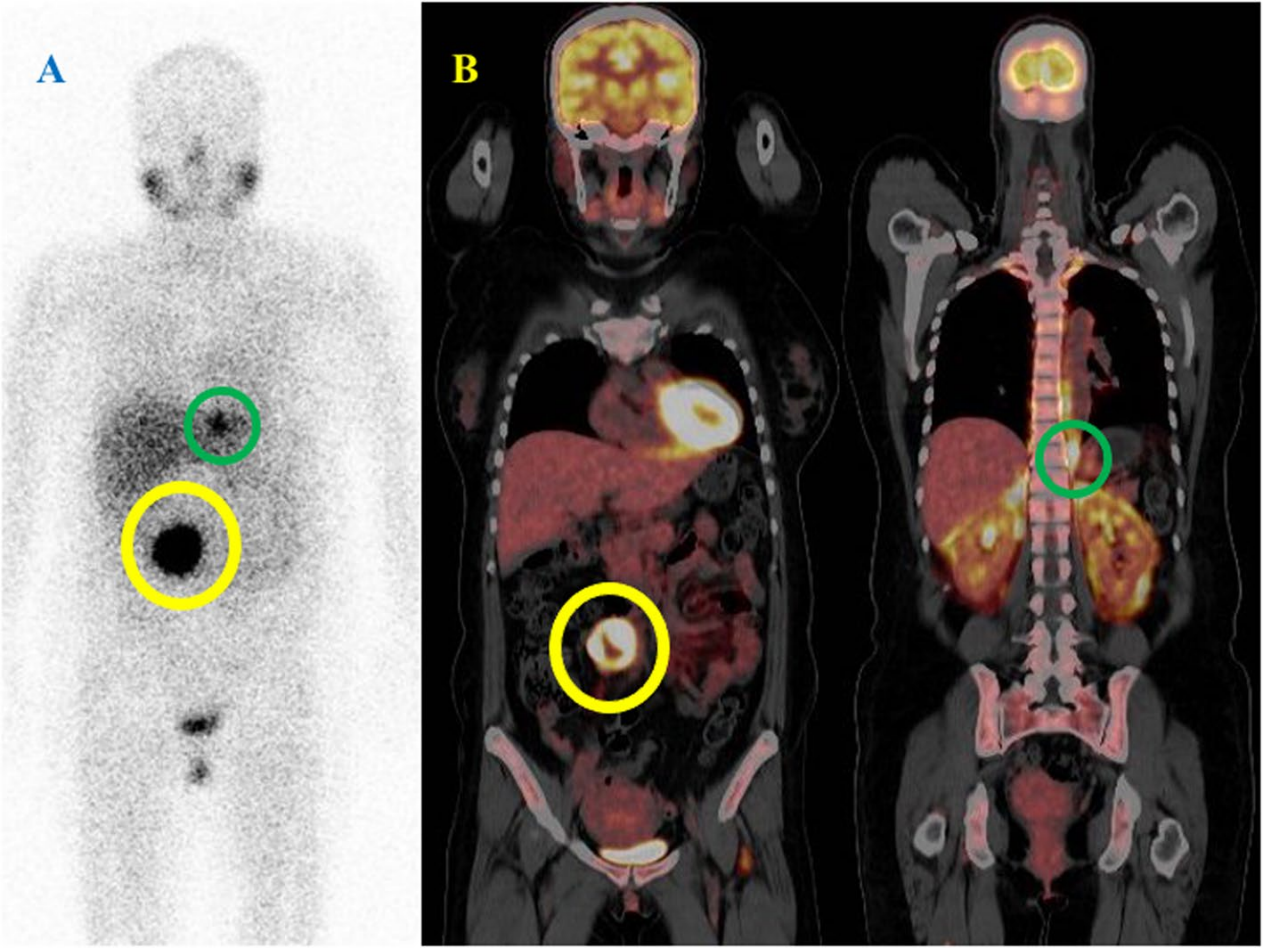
^18^F-FDG-PET and MIBG scintigraphy in paraganglioma with SDHB gene mutation. Thirty-seven y.o. female of paraganglioma with SDHB gene mutation. (A) ^123^I-MIBG scintigraphy showed positive accumulation in right retroperitoneal cavity (yellow circle) and left retroperitoneal cavity (green circle). (B) ^18^F-FDG-PET showed SUVmax:24.2 in right retroperitoneal cavity (yellow circle) and SUVmax:17.8 in left retroperitoneal cavity (green circle). Both tumors had PASS Score ≥ 4 and GAPP Score with poorly differentiation
